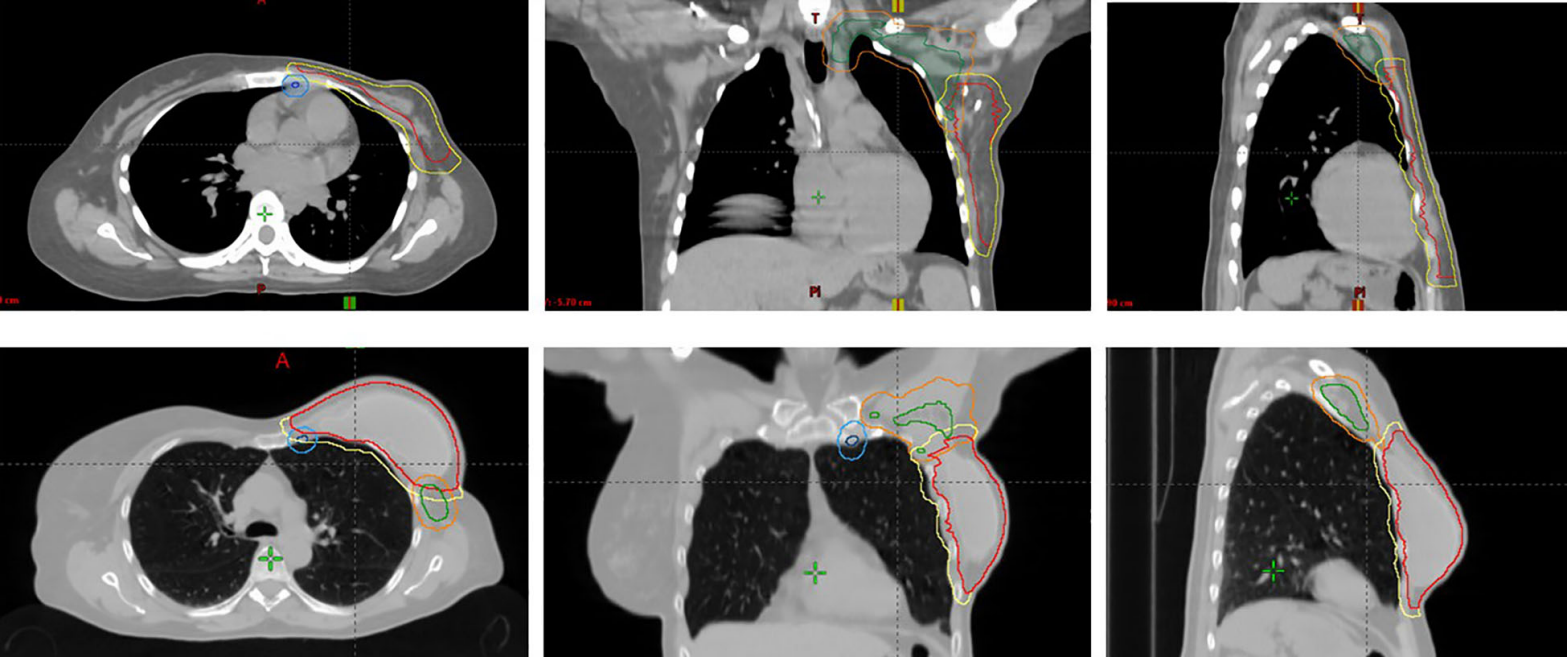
Two examples of patient CT images in axial (left), coronal (center), and sagittal (right) views showing PTV breast/chestwall (yellow), PTV_IMC (light blue), PTV_nodes (orange), CTV breast/chestwall (red), CTV_IMC (dark blue) and CTV_nodes (green).
